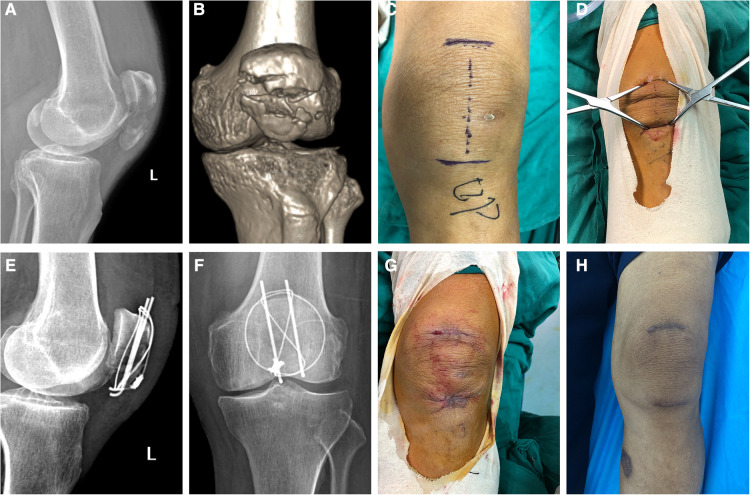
Radiographs and photographs of the knee of a 73-year-old male patient who suffered patellar fracture and received surgical treatment via the minimally invasive technique. (A) Lateral view of a preoperative radiograph of the knee joint. (B) 3D reconstruction image of patellar computer tomography scanning. (C) Photograph of an incision mark. (D) Two-pointed bone reduction forceps were applied to reduce the fracture displacement. (E) Lateral view of a postoperative radiograph of the knee joint reveals good bone reduction. (F) Anteroposterior view of a postoperative radiograph of the knee joint. (G) Photograph of an incision taken immediately after surgery. (H) Photograph of an incision taken in the third month after surgery.
